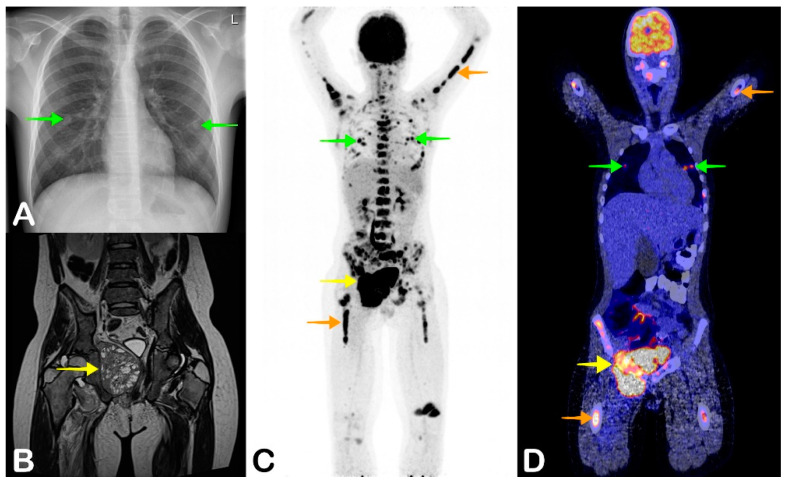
A 13-year-old boy presented to the hospital with a fever of three weeks, weight loss, and general malaise. He also had nonspecific pain in his legs and back and complained of nausea and vomiting. On physical examination, hepatomegaly was noticed. Laboratory testing showed normocytic anemia with a hemoglobin level of 5.2 mmol/L, leukopenia with a leukocyte count of 0.8 × 10^9^/L, and elevated lactate dehydrogenase of 300 U/L. Abdominal ultrasonography confirmed the hepatomegaly, but showed no clear cause of the fever. A chest X-ray showed multiple pulmonary nodules (A, green arrows), suggestive of metastases of an unknown primary tumor. Because the patient had multiple nonguiding symptoms, FDG-PET/CT was performed. Coronal maximum intensity projection FDG-PET and fused FDG-PET/CT showed a large FDG-avid mass in the right lesser pelvis (C and D, yellow arrow), multiple pulmonary metastases (C and D, green arrows), and focally FDG avid bone marrow (C and D, orange arrows). Biopsy confirmed the diagnosis of metastasized Ewing sarcoma. MRI was performed before treatment was started, which also showed the primary site of the Ewing sarcoma in the right side of the pelvis (B, yellow arrow). After chemotherapy, radiotherapy, and an autologous stem cell transplant, the patient recovered and remained in complete remission for five years of follow-up.
